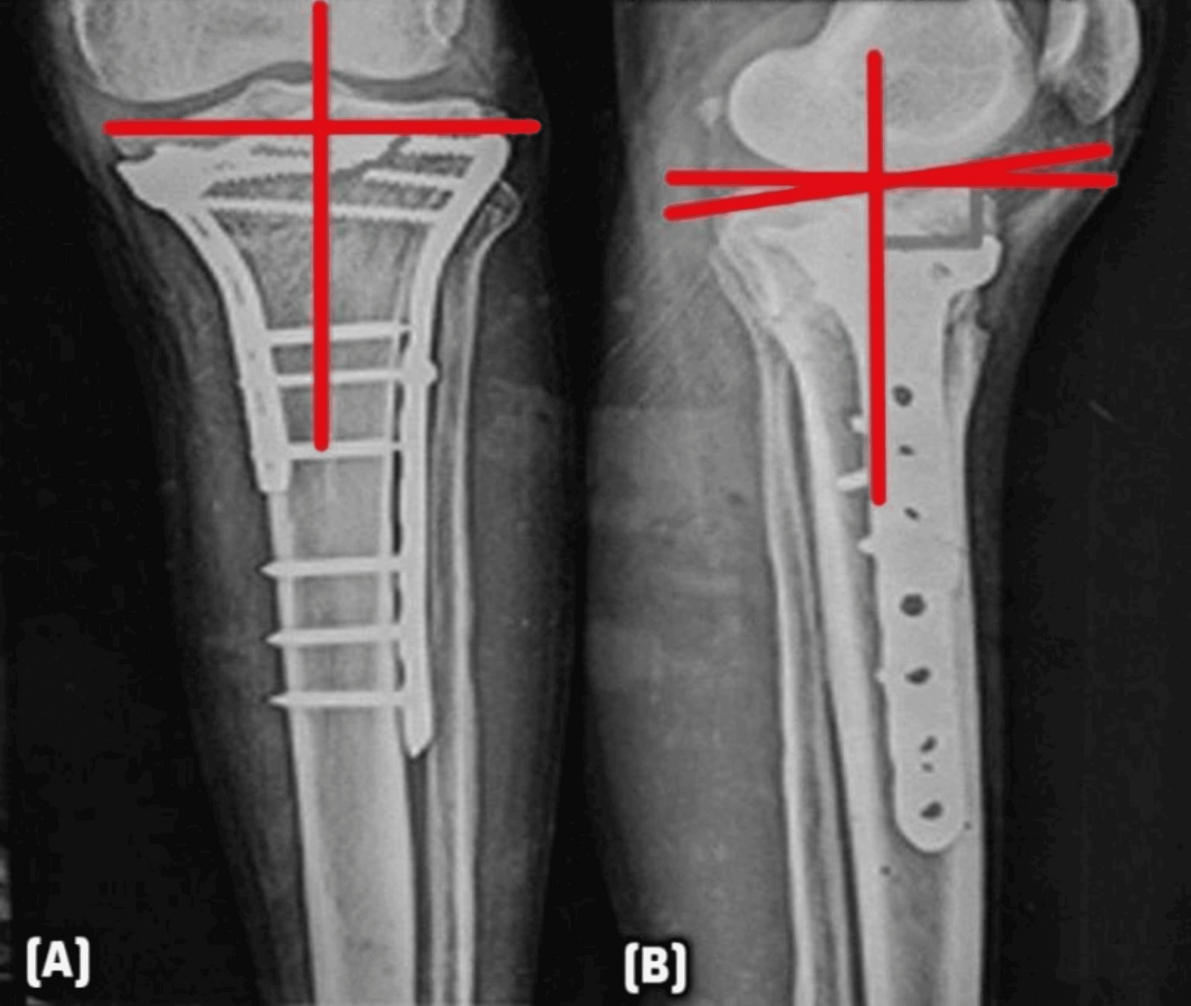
Radiographic assessment of postoperative alignment using MPTA and PPTA (A) Postoperative AP radiograph showing the MPTA measured between the tibial mechanical axis and the articular surface of the proximal tibia. The observed angle is within the normal range of 82°-92°. (B) Lateral radiograph showing the PPTA, formed between the tibial shaft and the tibial plateau, with a normal reference range of 4°-14°. These angles help assess coronal and sagittal alignment after surgical fixation of proximal tibial fractures. MPTA: medial proximal tibial angle; PPTA: posterior proximal tibial angle; AP: anteroposterior
